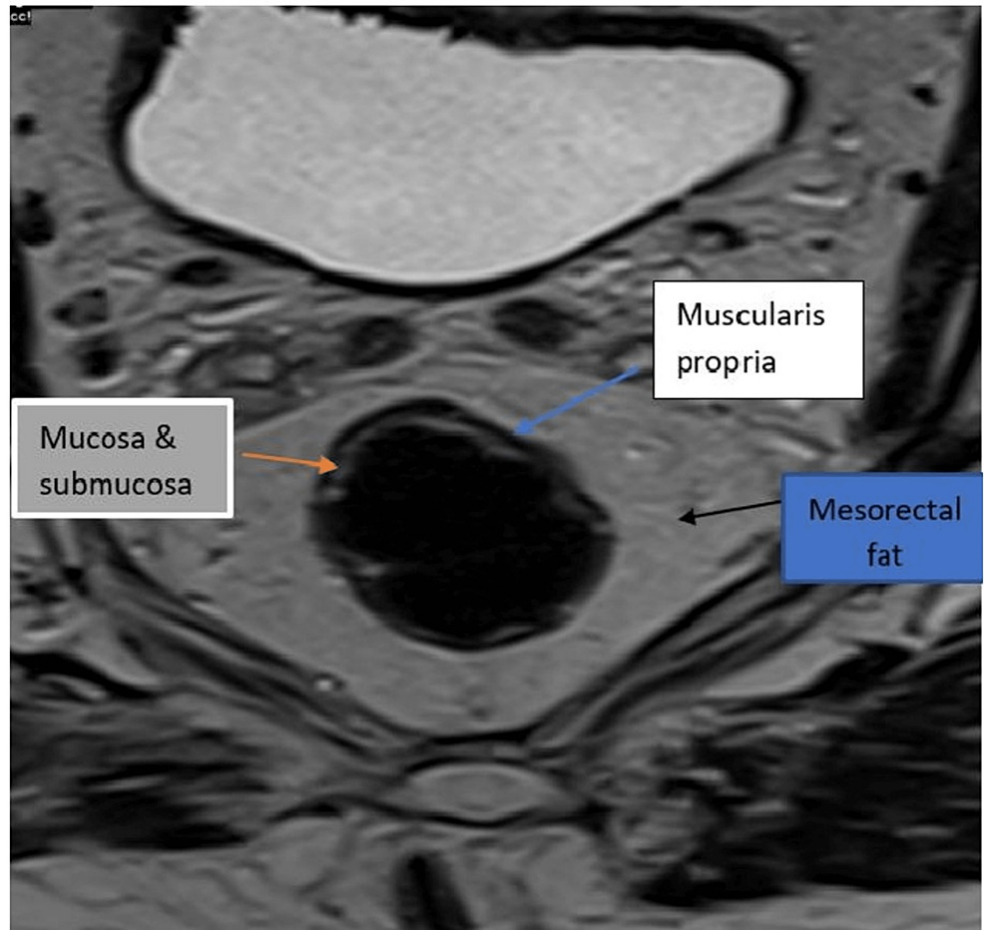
Axial T2-weighted MRI image of the rectum showing the rectal wall and surrounding mesorectum MRI: magnetic resonance imaging. Created by Collins O. Opara.
